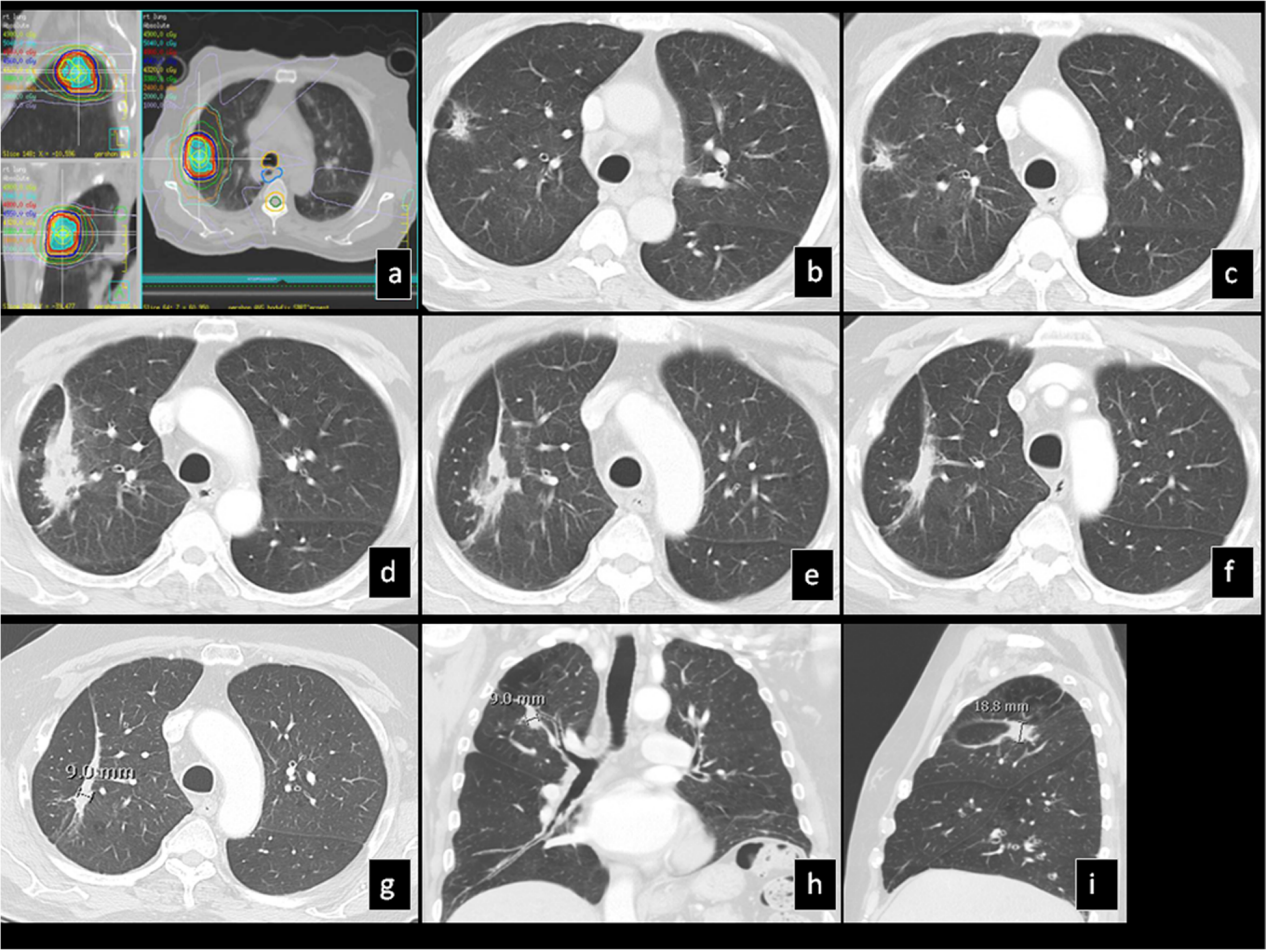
SBRT-treated lung cancer demonstrates stabilization at 35 months: a) Isodose multiplanar images for planning. b) Pre-SBRT CT shows a part-solid nodule. c) The nodule slightly decreased in size 9 months post-SBRT. d) 13 mo post-SBRT, the overall volume of the surrounding radiation changes obscuring the nodule increased in size with a pattern resembling “peribronchial consolidation”. e) 23 mo post-SBRT, the radiation changes decreased in size demonstrating a “scar-like” pattern and f) in 35 mo, there was stabilization of the “scar-like” pattern. g) 48 mo post-SBRT there was no change. Coronal (h) and sagittal (i) reconstruction at 48 mo (same time point as in fig. 5f) shows “scar-like pattern” after stabilization. The “width” of the lesion is small in 2 of the 3 planes (axial (g) and coronal (h)) compared to the third plane (sagittal (i)). To correctly identify the “scar-like pattern” careful evaluation of all 3 planes is needed as the lesion usually looks “bulkier” in one plane giving the false impression of a more “mass-like” pattern
